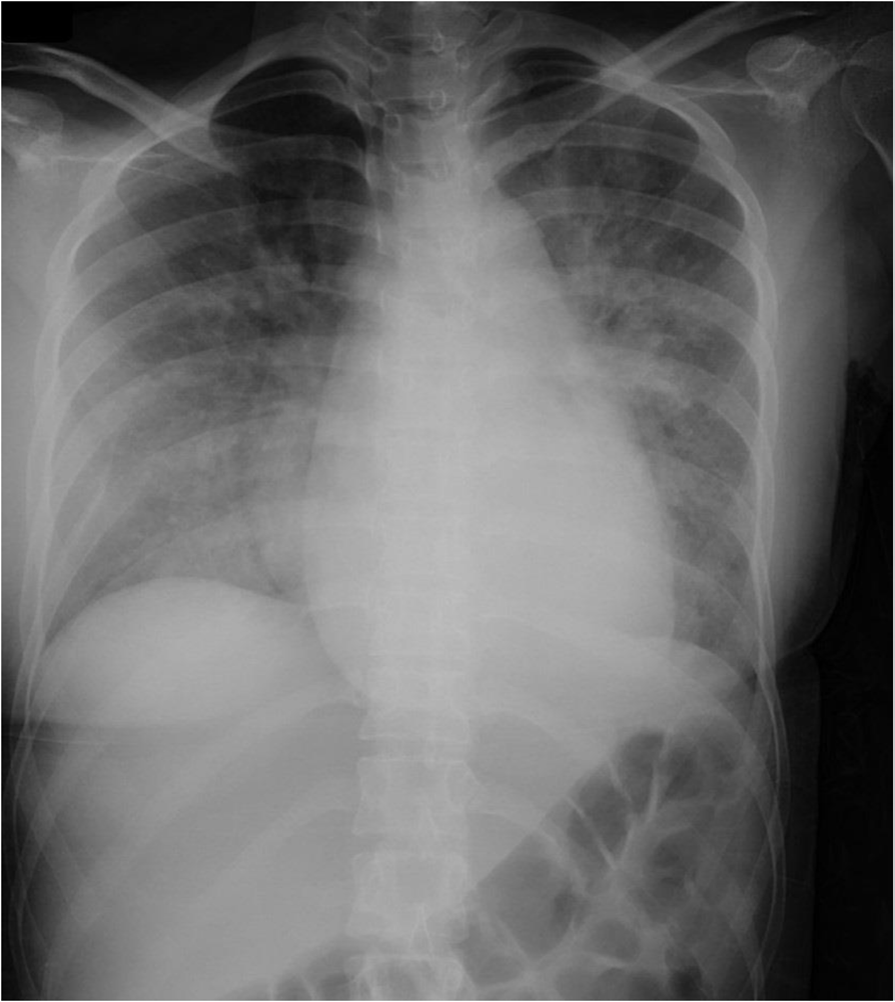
Chest X-ray on the day of cesarean section. The image demonstrates the enhancement of pulmonary vessels
